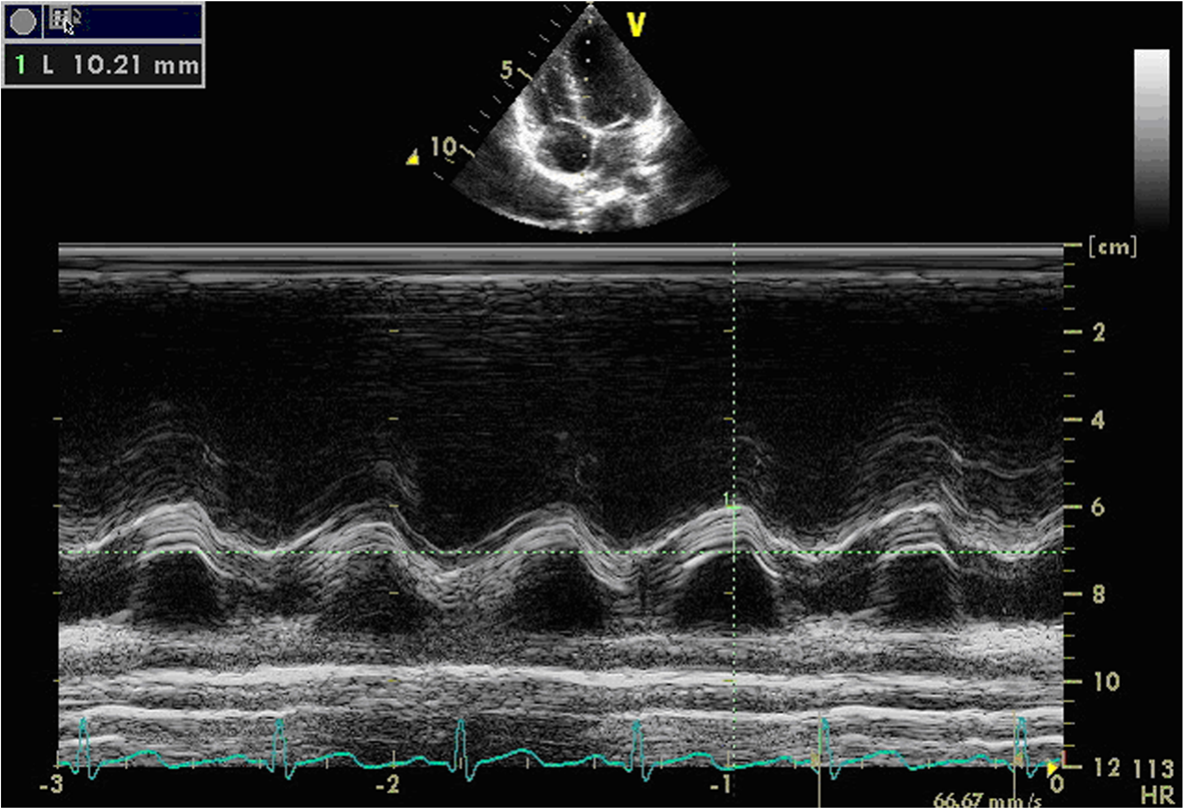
TAD measured from the interventricular septum (TADs). The upper image was the apical 4-chamber view, the lower curve was the M-mode line of the interventricular septum. TADs was the distance measured from the highest to the lowest of the line (in the above image).
